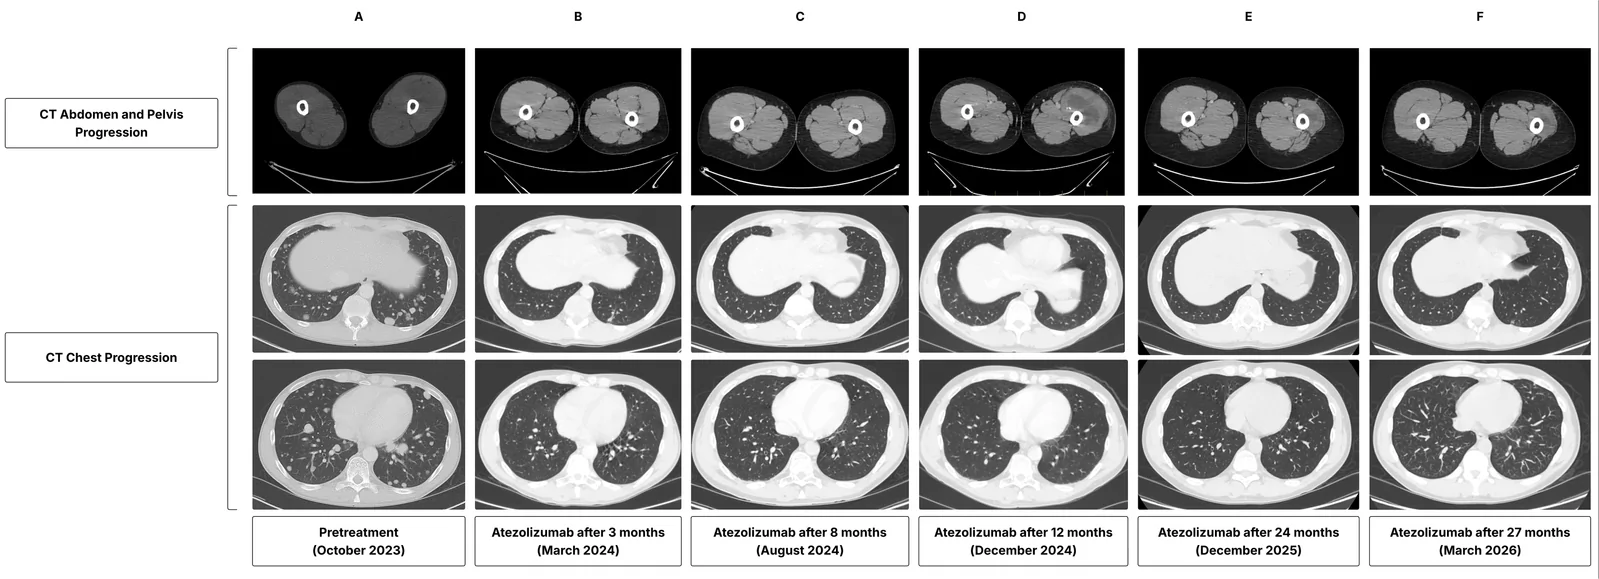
CT imaging demonstrating treatment response over time. Axial CT images demonstrating atezolizumab treatment response over time across the primary and metastatic lesions. The top row shows abdomen and pelvis CT at the level of the thighs that was obtained to monitor the primary lesion and screen for extrapulmonary and extrathoracic disease. The middle and bottom rows show chest CT demonstrating reduction of pulmonary metastatic disease. (A) Pre-treatment baseline imaging obtained at an outside institution in October 2023, demonstrating innumerable bilateral pulmonary metastases. (B) Chest CT in March 2024, 3 months after initiation of atezolizumab, demonstrating marked interval reduction in the number and size of pulmonary metastases. Almost all nodules completely resolved, and only a few millimetric micronodules remained. (C) Chest CT in August 2024, 8 months after initiation of atezolizumab, demonstrating further regression of residual micronodules. (D) Chest CT in December 2024, 12 months after initiation of atezolizumab, demonstrating stable residual micronodules without evidence of progression or new lesions. (E) Chest CT in December 2025, 24 months after initiation of atezolizumab, demonstrating continued absence of measurable pulmonary metastatic disease. (F) Chest CT in March 2026, 27 months after initiation of atezolizumab, demonstrating sustained near-complete response. Throughout follow-up, abdomen and pelvis CT showed no evidence of extrapulmonary or extrathoracic metastatic disease. CT, computed tomography.
